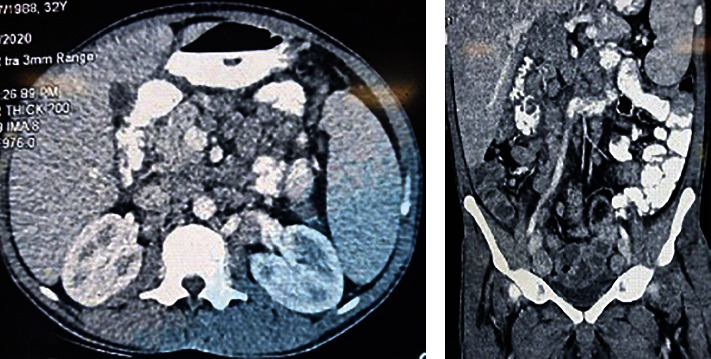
(a) Axial view of CT scan of the abdomen showing hepatosplenomegaly with pre- and para-aortic mesenteric lymphadenopathy; (b) enlarged liver and spleen with multiple intra-abdominal lymphadenopathies.
